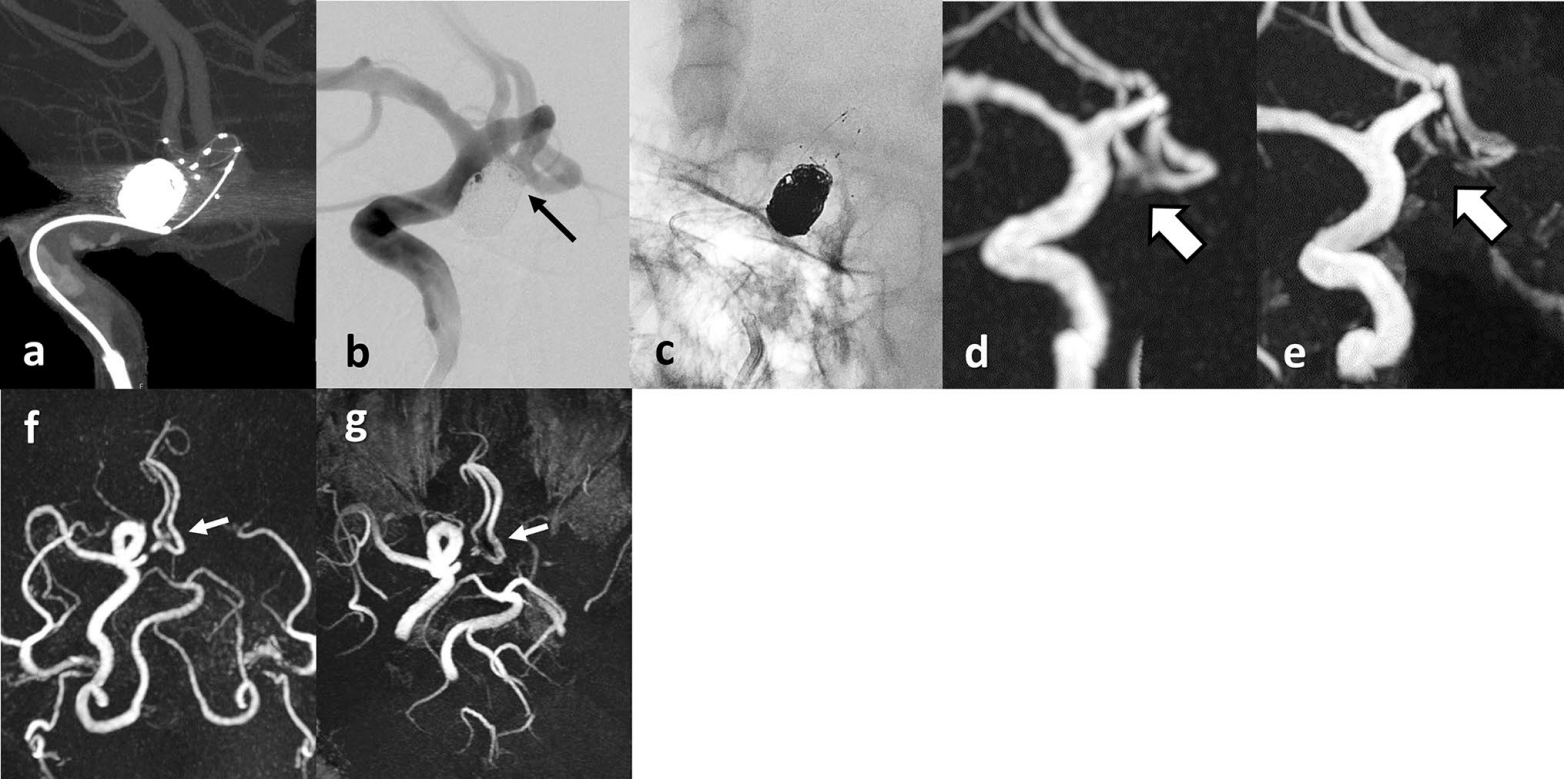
A 66-year-old male patient with a recurrent unruptured AcomA coiled aneurysm (Case 4). a CBCT after PulseRider placement (T shape, 10 mm, 2.7–3.5 mm). b Postoperative DSA image after coil embolization with PulseRider. The black arrow indicates a small neck remnant. c Postoperative native image after coil embolization with PulseRider. d Silent MRA image clearly shows a small neck remnant and parent artery in the bifurcation (white arrow). e TOF-MRA failed to depict the area signal shown by the PulseRider device (white arrow). f The entire image of silent MRA (axial view). A small neck remnant was visualized (white arrow). g The entire image of TOF-MRA (axial view). The signal around the aneurysmal neck was not detected (white arrow). *AcomA* anterior communicating artery, *CBCT* cone-beam computed tomography, *DSA* digital subtraction angiography, *MRA* magnetic resonance angiography, *TOF* time-of-flight
